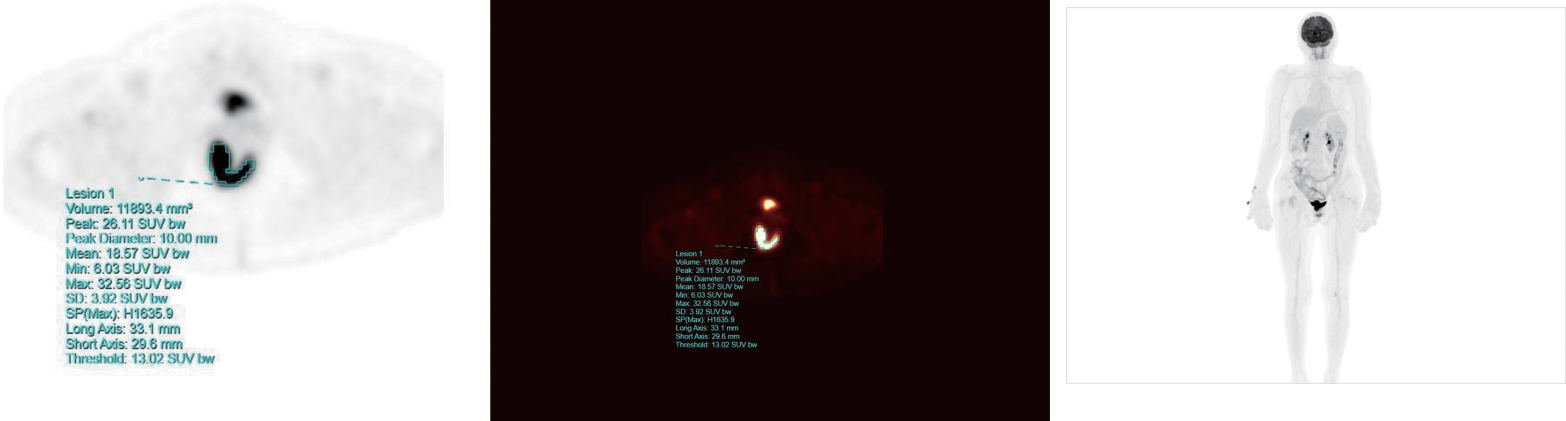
PET-CT image analysis and metabolic information calculation.
